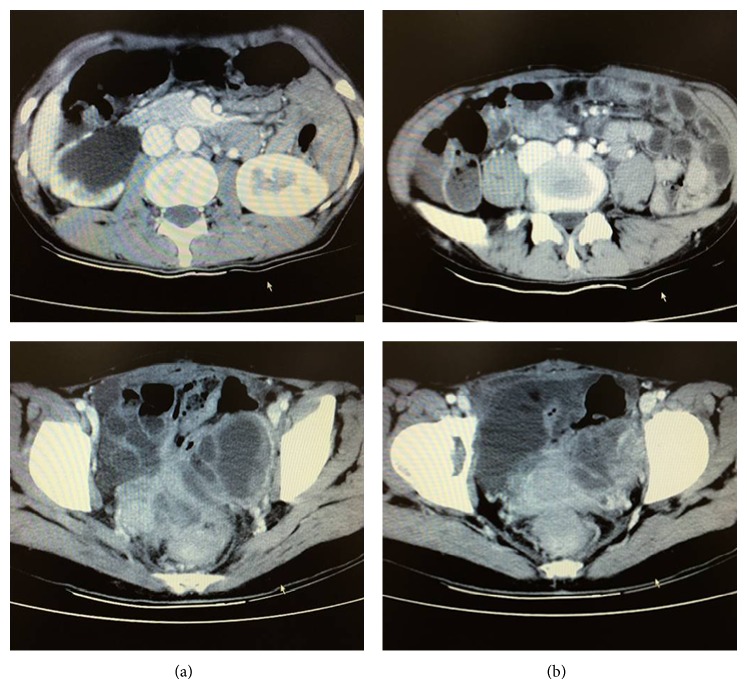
Abdominal and pelvic computed tomographic scan demonstrated thickening of rectal wall with inhomogeneous enhancement and a 4 cm soft tissue mass that involved the rectum and left ovary. Right hydronephrosis and hydroureter were also revealed.
